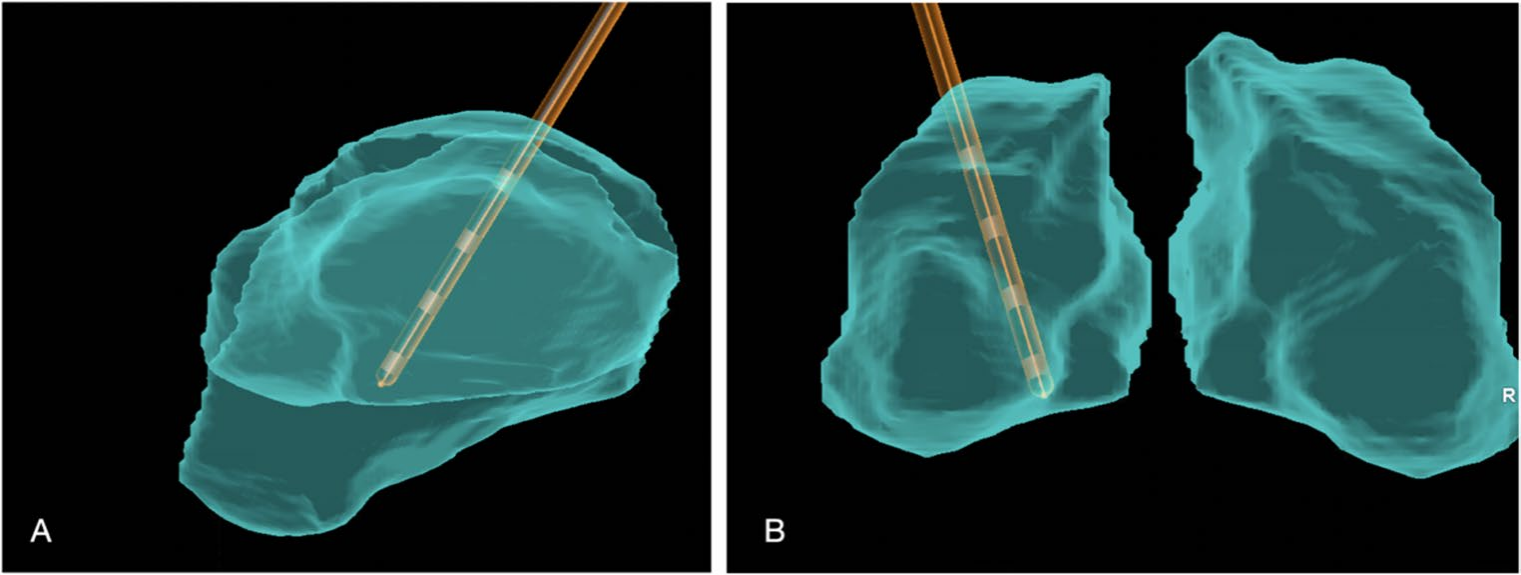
Post-operative reconstruction based on the fusion of CT scan to the MRI, using BrainLab Suite v3.4, showing the left pulvinar lead into the thalamus (in blue). The lead contacts are spaced by 3.5 mm
